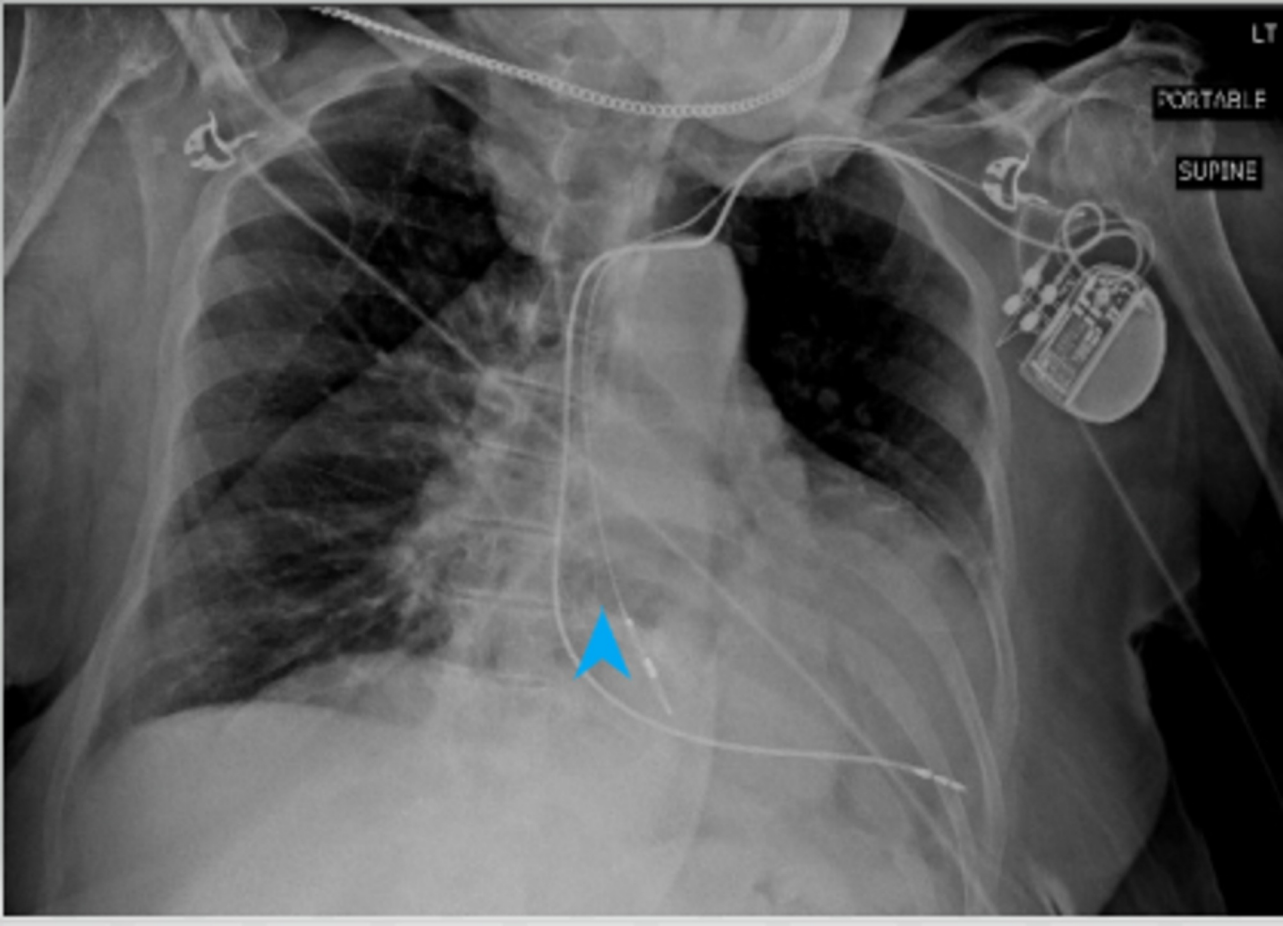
Anteroposterior view chest X-ray. The arrowhead pointing at the gastric shadow in the thoracic cavity.
